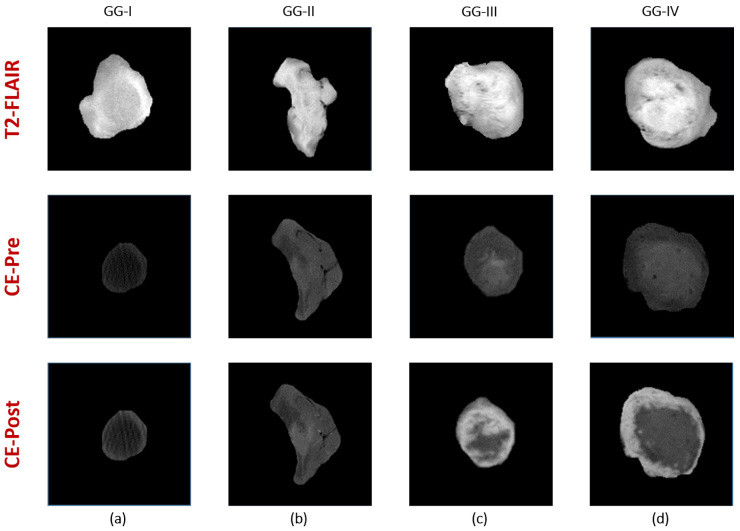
Visualization of texture differences between four gliomas with different grades (a) Grade I, (b) Grade II, (c) Grade III, and (d) Grade IV using contrast-enhanced T1-MR (pre and post phases) and T2-MR (FLAIR).
